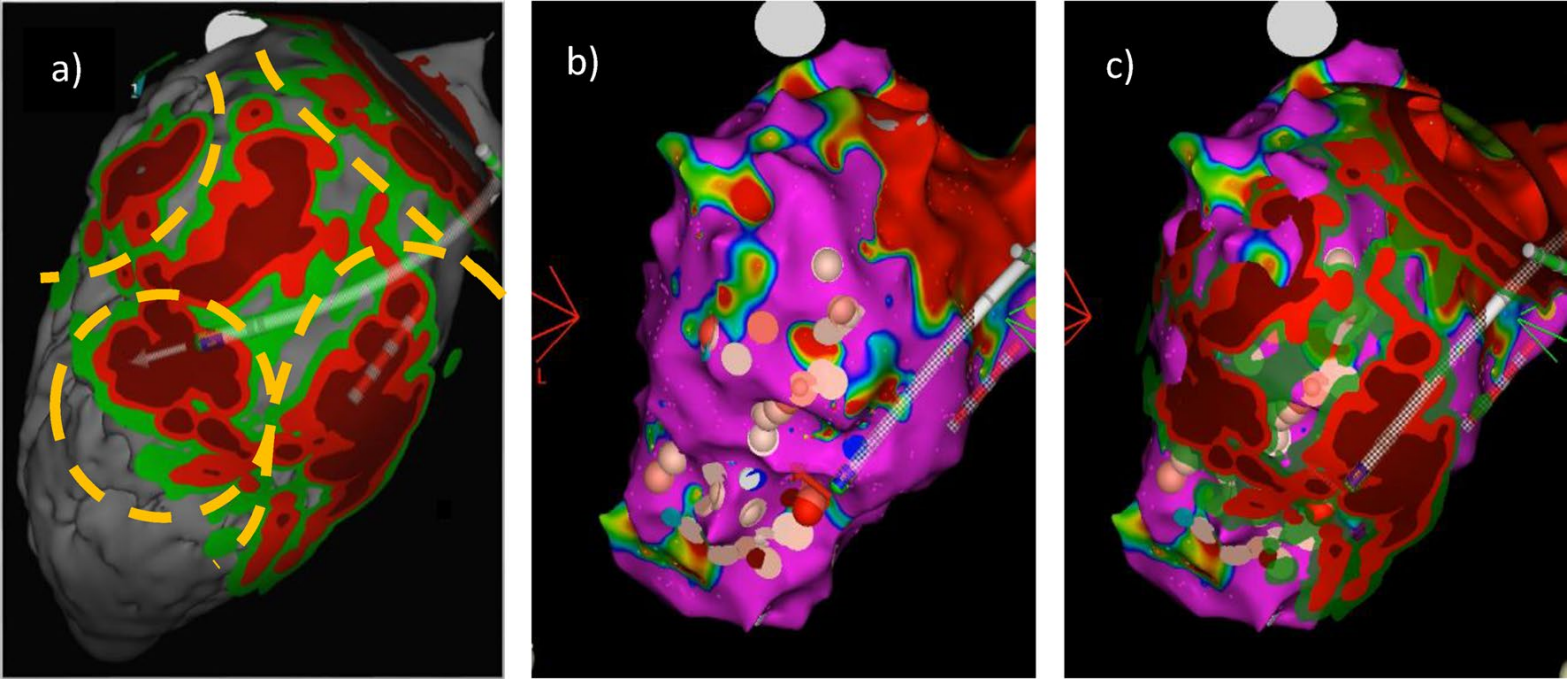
Inferior views on the left ventricle during an ep study of a dilative cardiomyopathy patient with recurrent ventricular tachycardias post myocarditis. The images A–C show corresponding views on the InHeart segmentation (a), the CARTO3 voltage mapping (b), and the merged inHeart/Voltage map (c). The color gradient segmentation in a) represents wall thinning and thereby the extent of left ventricular scar (gray: endocardium, green: scar as detected in late-enhancement CT, red: 2 mm, brown: 1 mm wall thickness). The dashed yellow lines represent possible VT circuits as derived from scar segmentation. The voltage map (b) shows healthy endocardial myocardium without relevant abnormal electrograms or arrhythmogenic substrate. C) shows the fused imaging of (a) and (b)
